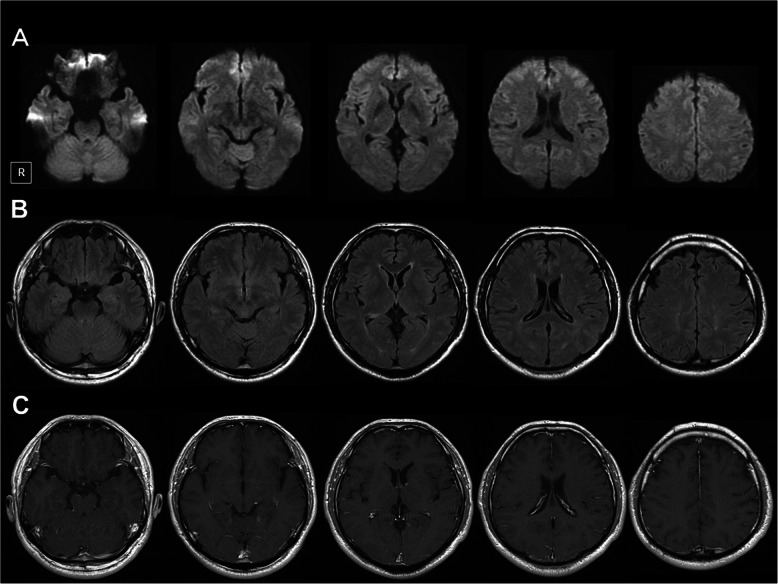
Brain magnetic resonance imaging on admission. There are no abnormal findings on (a) diffusion-weighted images, (b) fluid-attenuated inversion recovery, and (c) T1-weighted images with gadolinium enhancement
